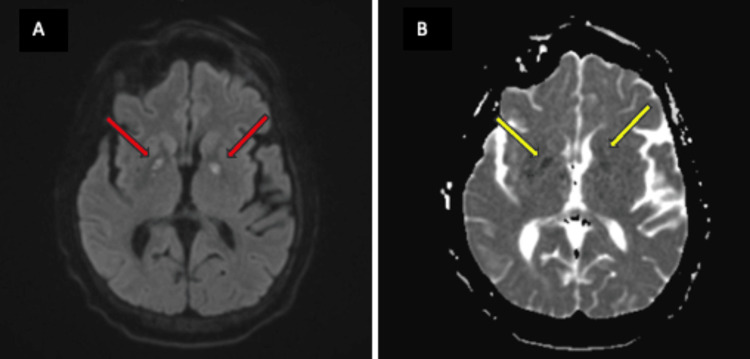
Symmetric bilateral globus pallidus on the brain MRI A: MRI-DWI hyperintensities (red arrows); B: MRI-ADC hypointensities (yellow arrows) MRI: magnetic resonance imaging; DWI: diffuse-weighted imaging; ADC: apparent diffusion coefficient
